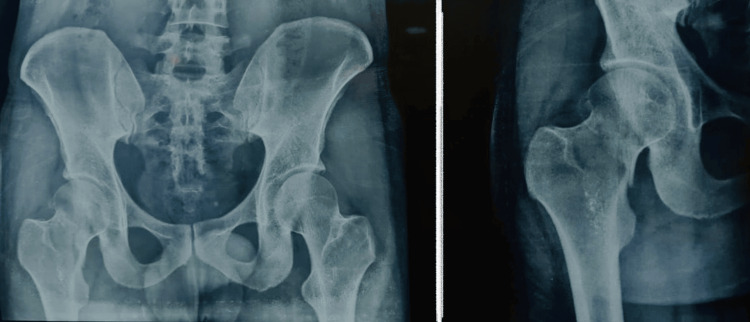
(Left panel) Pre-operative X-ray of the pelvis and both hips in an anteroposterior view. (Right panel) Close-up anteroposterior view of the right hip.
